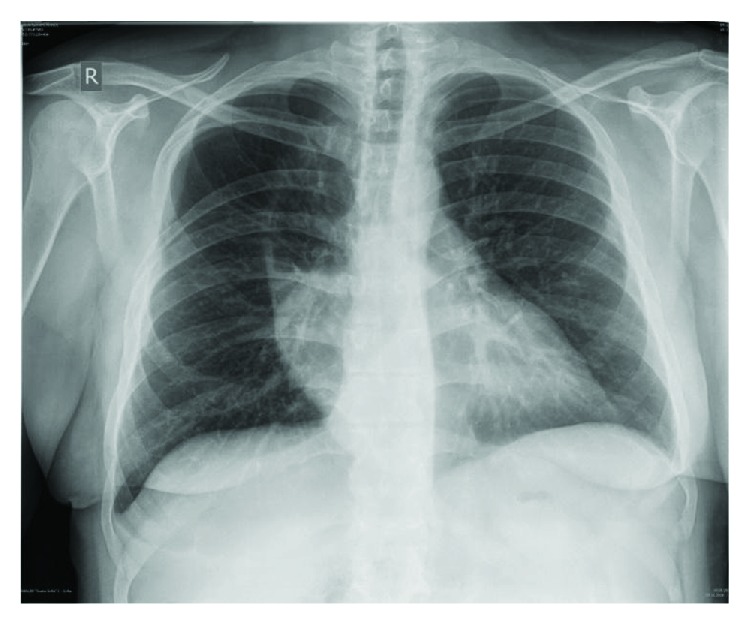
Chest X-ray after the decortication.
